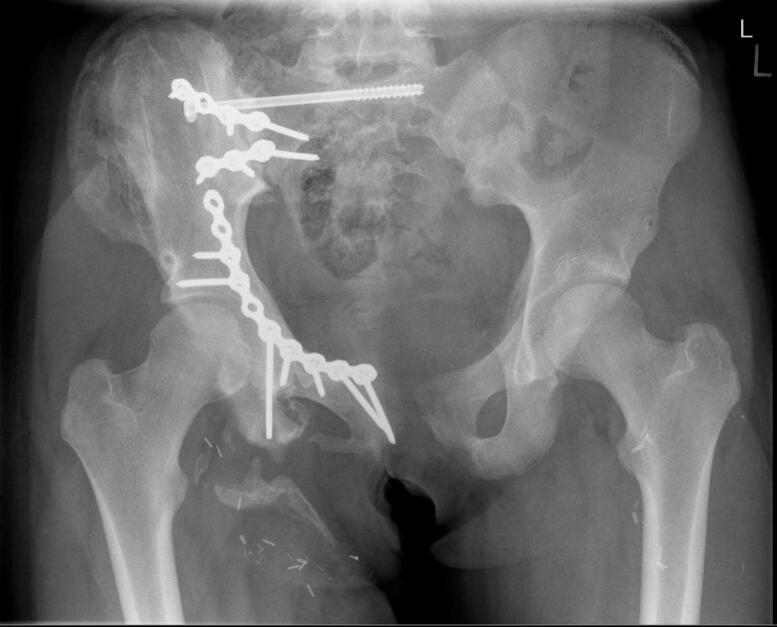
Anteroposterior Pelvic radiograph 6 weeks after Open reduction and internal fixation.
